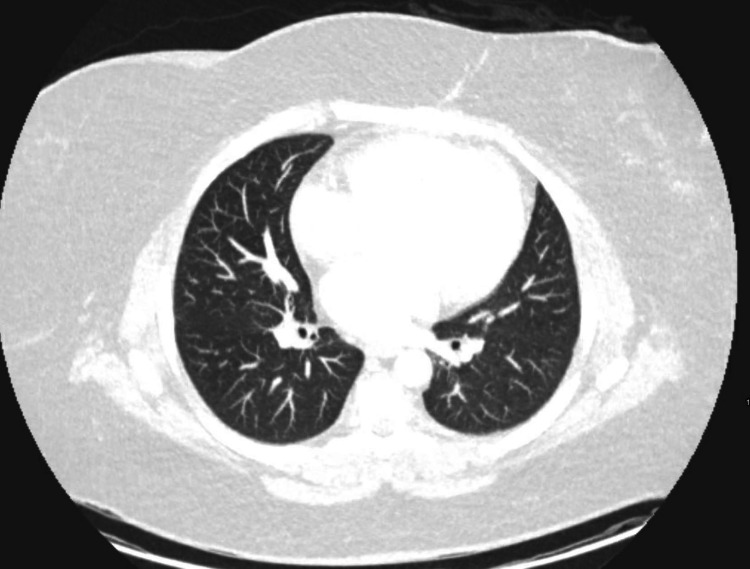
Transaxial chest CT with no evidence of pulmonary fungal disease. CT: computed tomography
